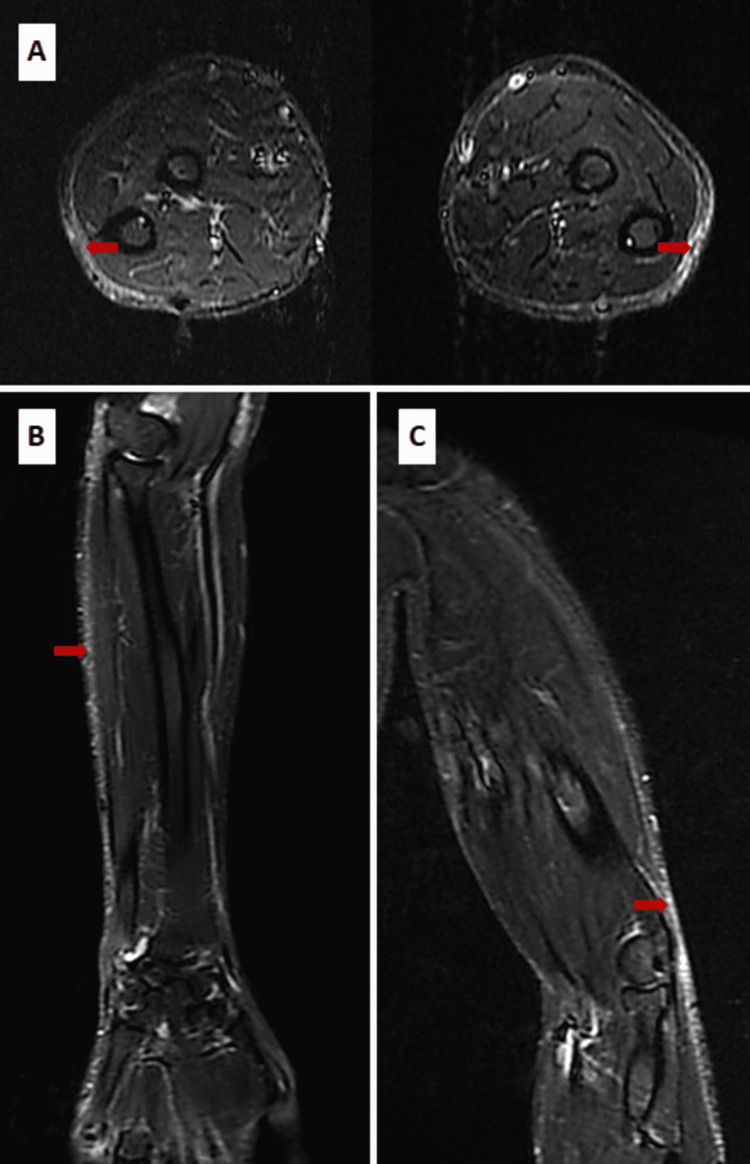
Fasciitis of the upper limbs on MRI Thickening and STIR hypersignal of the deep fasciae (arrows) without muscle damage. Symmetrical involvement of the forearms (A and B) and arms (C).
